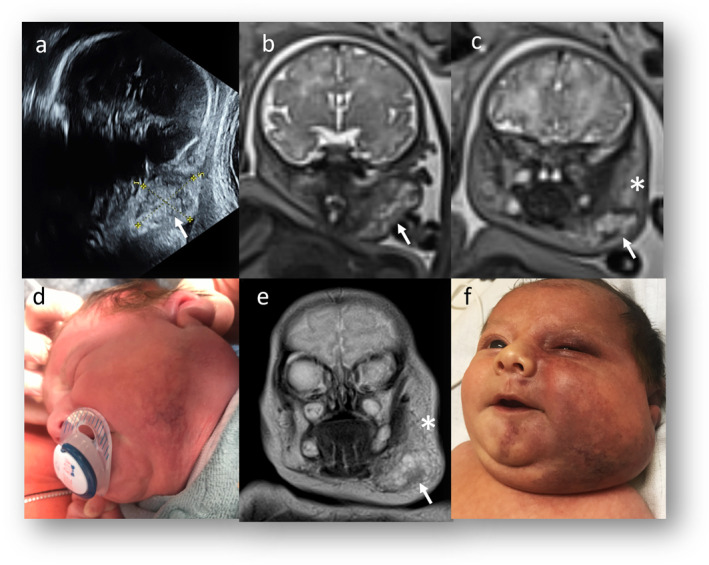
(a–f, Case 3). Coronal Doppler ultrasound at 33 weeks' gestation shows an echogenic infiltrative mass involving the left subcutaneous and retromandibular soft tissues (a, arrow). These findings correspond to the T2‐hyperintense infiltrative lesion seen on coronal fetal MRI (b, c, arrow), with discrete involvement of the left temporal soft tissues compared with the normal right side (c). Clinical examination at birth confirmed the prenatal imaging findings and demonstrated violaceous angiomatous discoloration of the overlying skin (d). Postnatal coronal T2‐weighted MRI showed stable discrete hyperintensity of the left submandibular and temporal infiltration (e, arrow). During the first two weeks of life, the infant developed significant clinical deterioration with increased facial infiltration and progressive violaceous discoloration, associated with thrombocytopenia (26 G/L), hypofibrinogenemia (1 g/L), and markedly elevated D‐dimer levels (> 20,000 ng/mL). KMP was diagnosed based on these characteristic findings, prompting the emergency initiation of combined sirolimus–steroid therapy (f).
